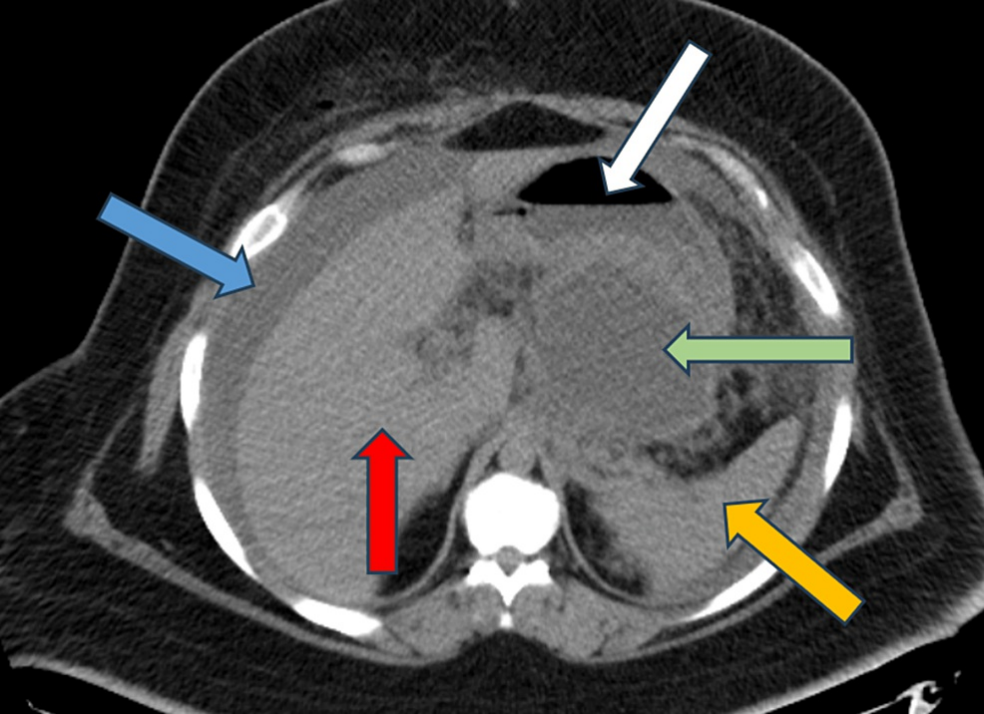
Axial cut of upper abdominal CT shows perihepatic free fluid (blue arrow), shrunken pseudocyst (green arrow), and stomach larger than before (white arrow). The spleen is depicted with an orange arrow, while liver is marked with a red arrow.
